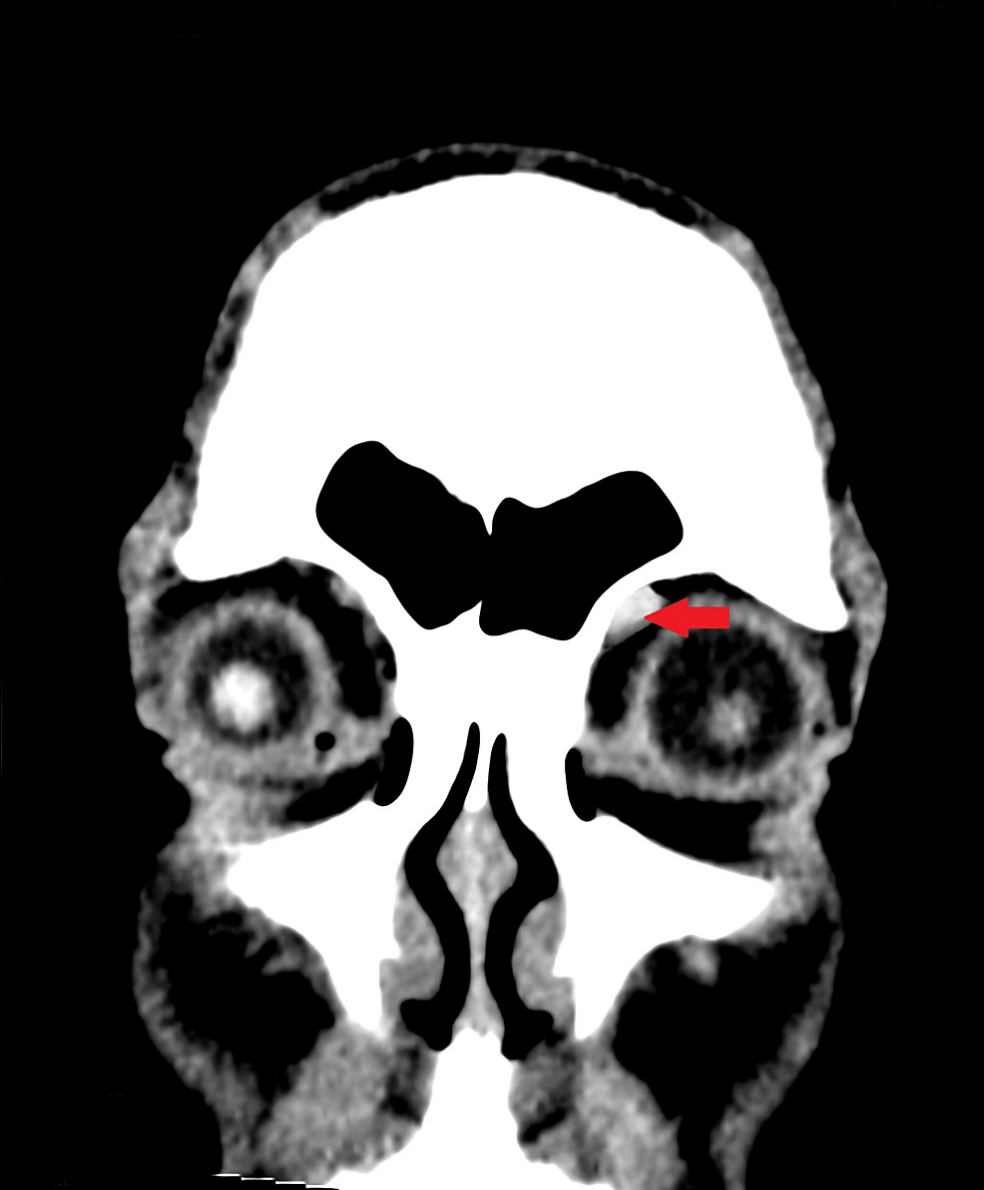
CT scan showing enlargement of the left superior oblique pulley and tendon (red arrow) CT, computerized topography
